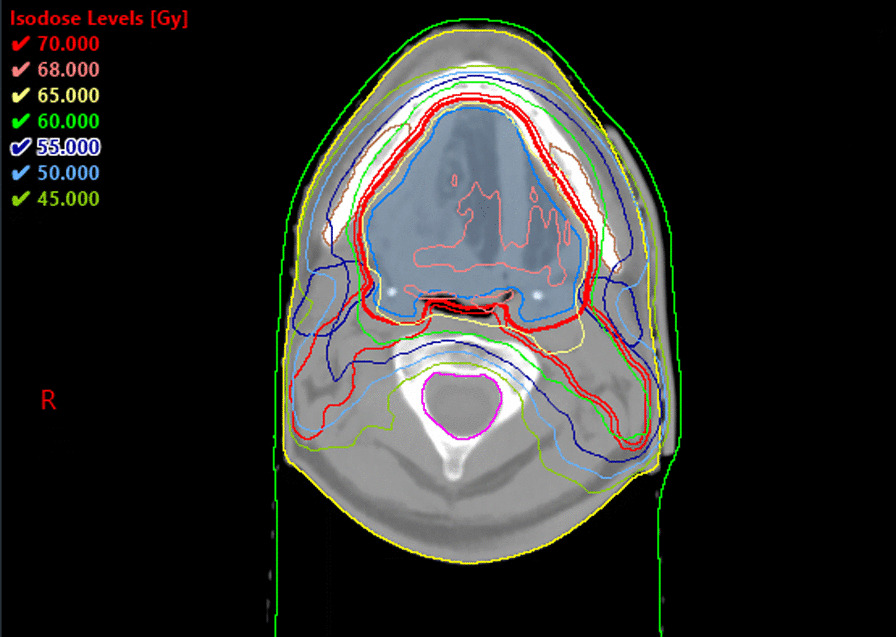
Conventional radiotherapy. Dose distribution shown on the CT scan. Isocenter plane of the 3rd stage of initial plan
